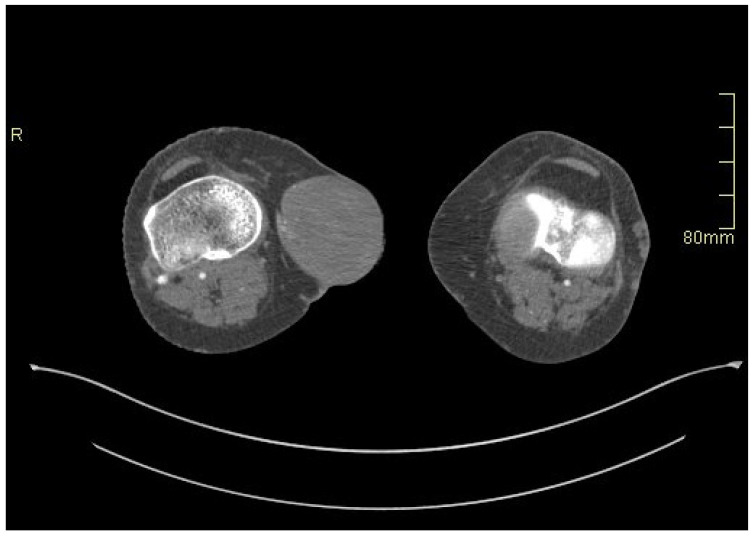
Great saphenous vein aneurysm on CTA.
